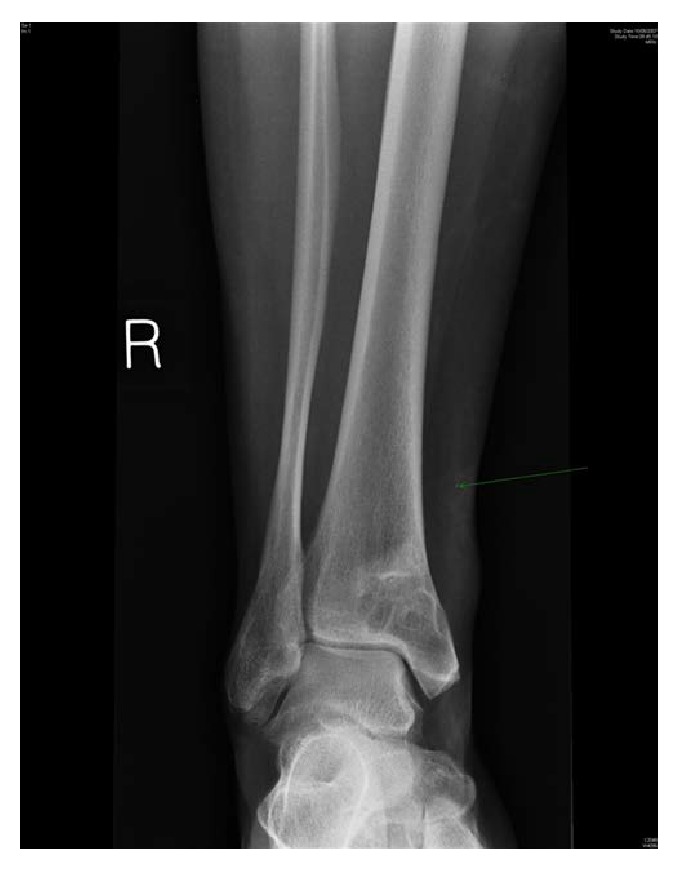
AP X-ray of ankle showing cystic lesion in distal tibia.
